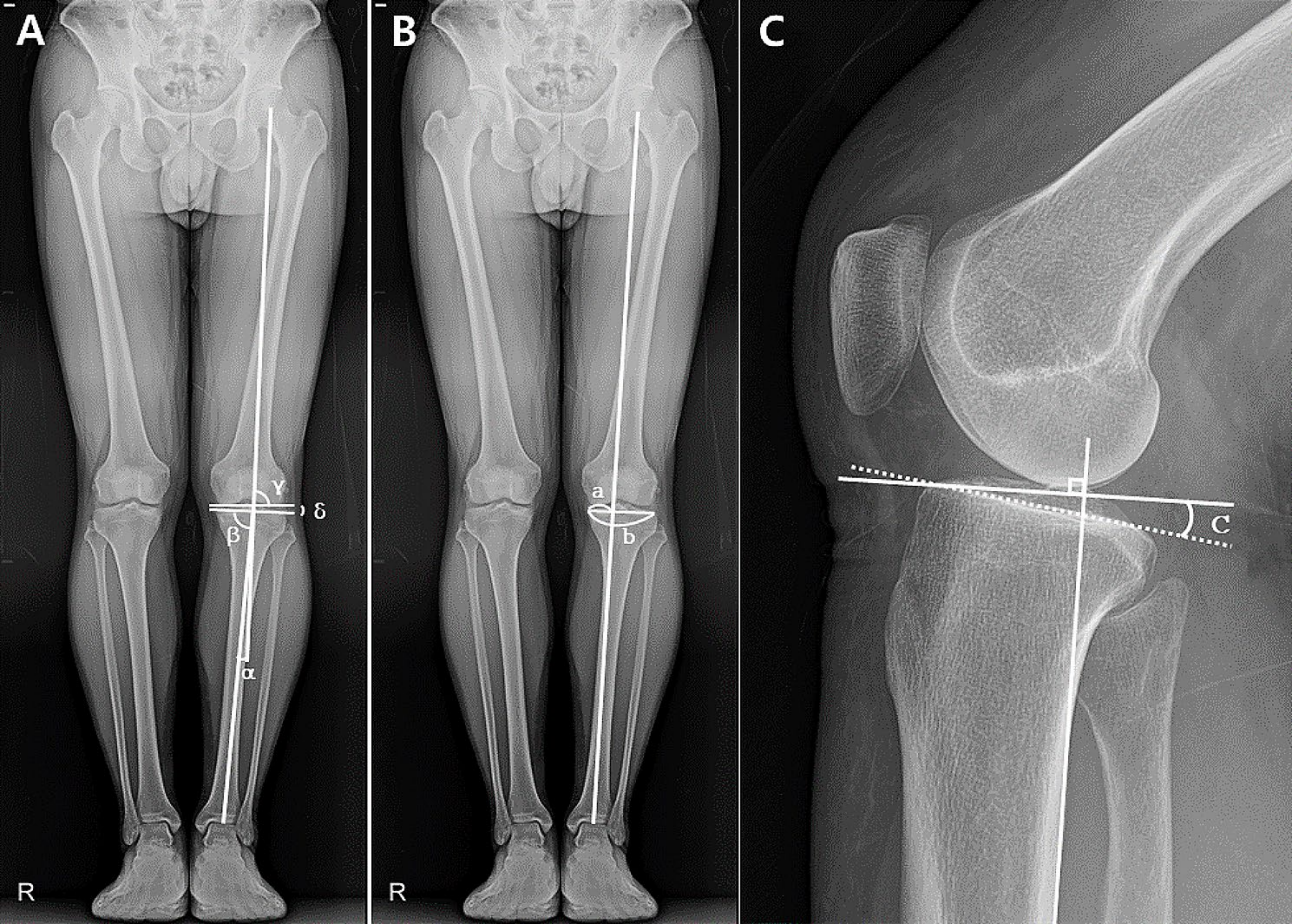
(A) Radiographic measurements of hip-knee-ankle angle (α, °), medial proximal tibial angle (β, °), lateral distal femoral angle (γ, °), and joint-line convergence angle (δ, °). (B) Radiographic measurement of weight-bearing line ratio (a/b x 100, %). (C) Radiographic measurement of posterior tibial slope (c, °)
